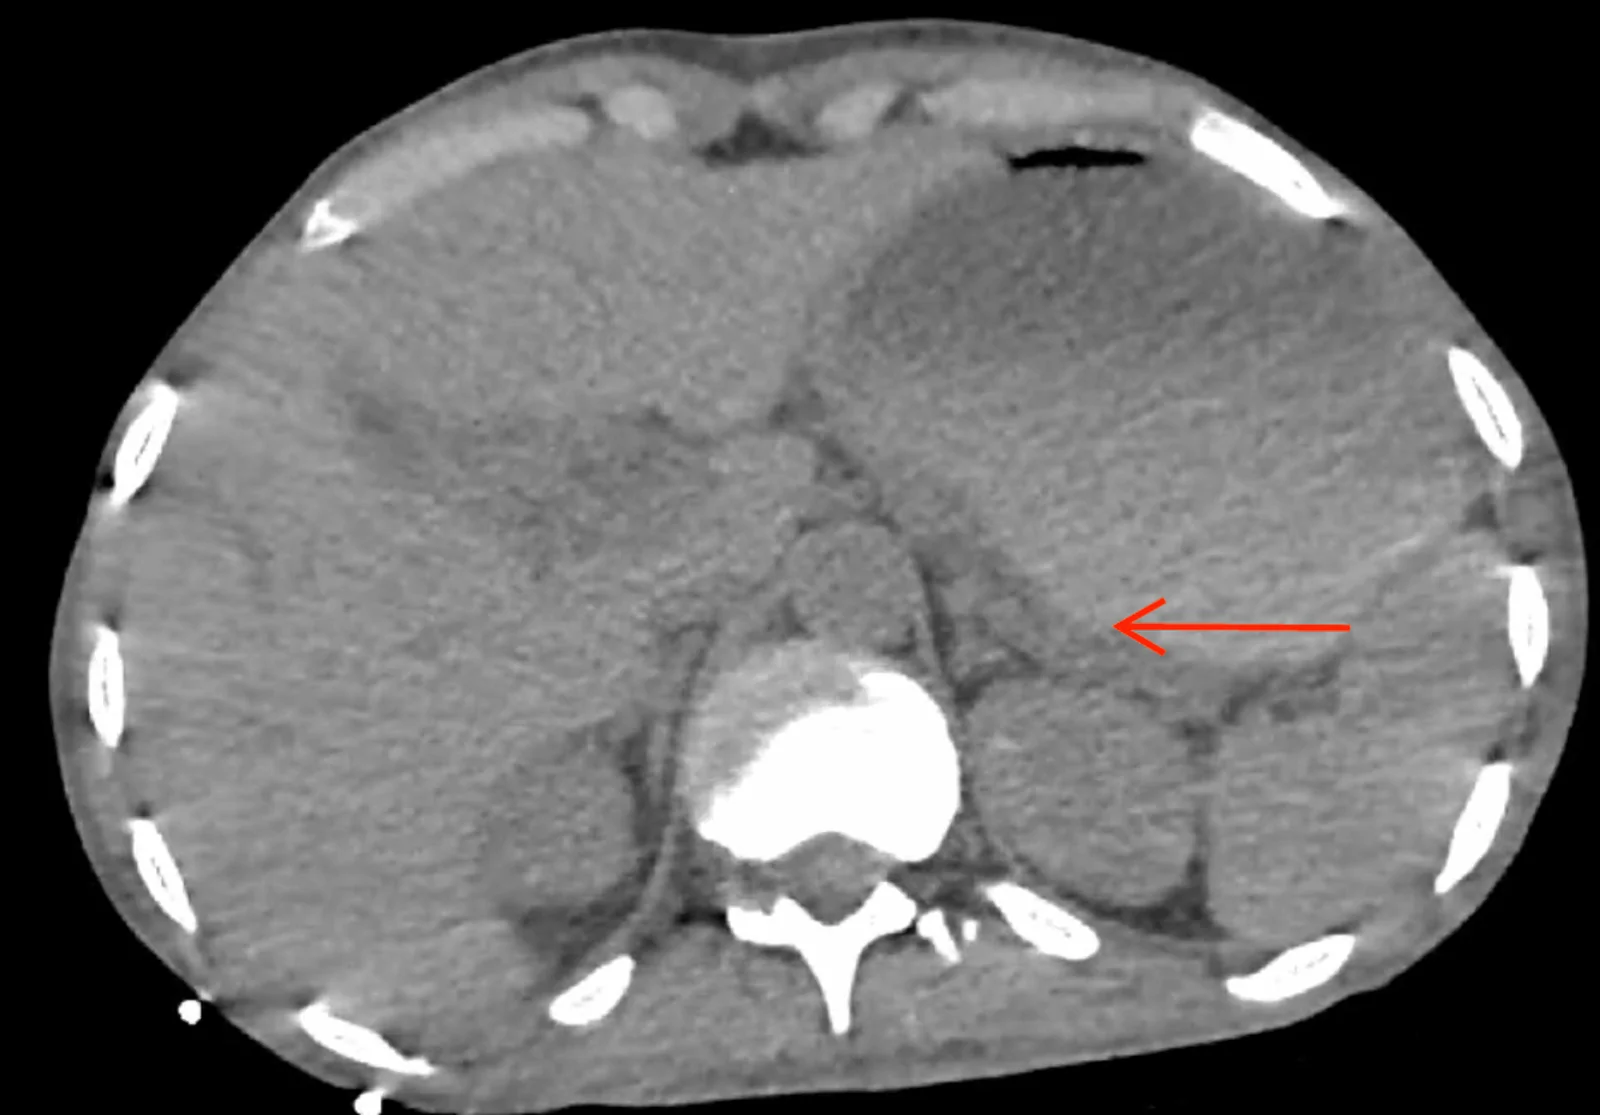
Non-contrast CT of the abdomen obtained 48 hours after admission demonstrating resolution of previously identified gastric pneumatosis Arrow depicts the resolution of gastric free air.
